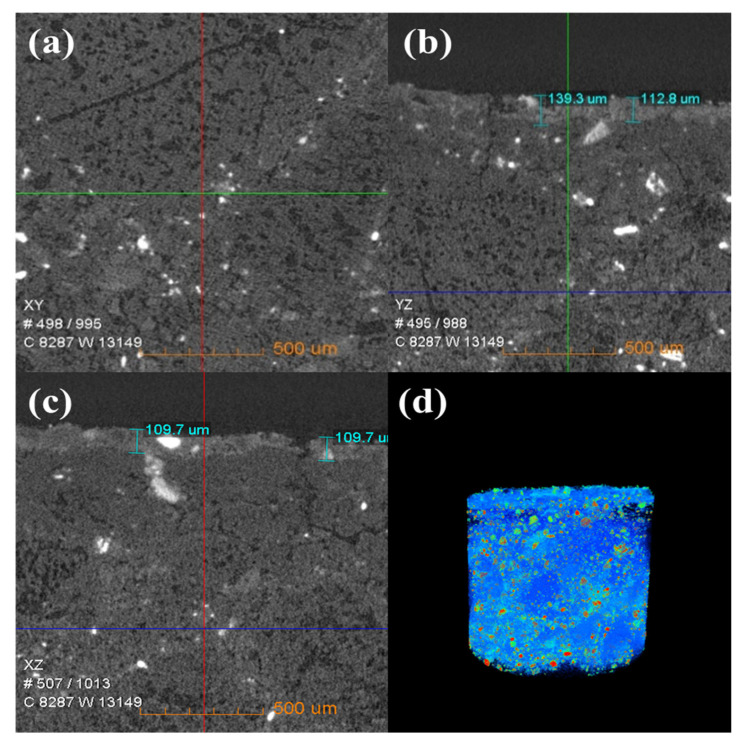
X-ray CT of a waste saggar sample. (a), (b), and (c) Internal X-ray CT figures of a waste saggar sample scanned from the XY, YZ, and XZ directions, respectively. (d) Three-dimensional image in which bright and dark colors represent high and low density, respectively.
